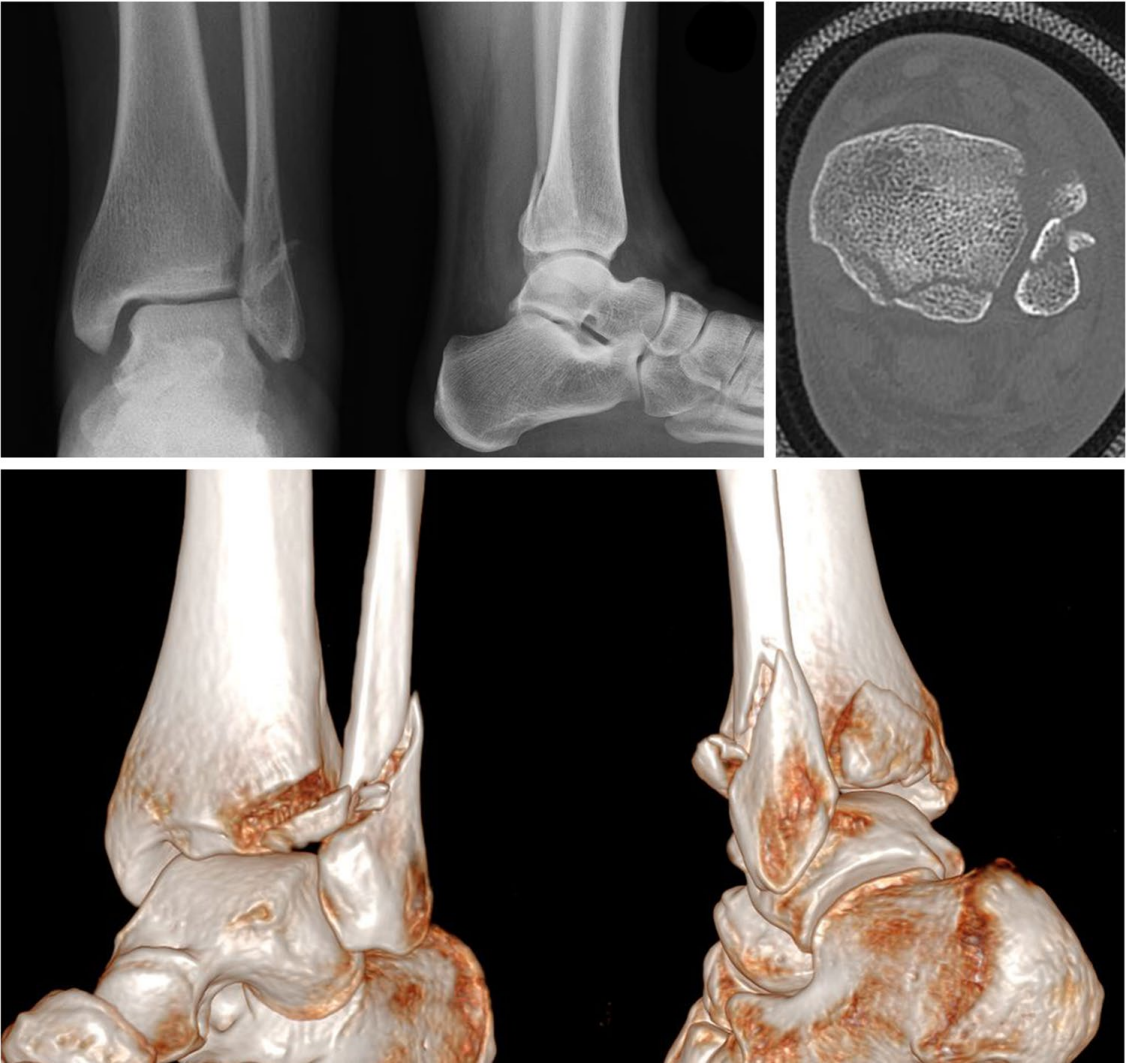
Standard X-rays and CT-scan showing a short oblique lateral malleolus fracture with anterior comminution, an avulsion fracture of the Chaput tubercle, and a displaced and comminuted fracture of the posterior malleolus Haraguchi 1/Bartonìček 2 (combination C)
